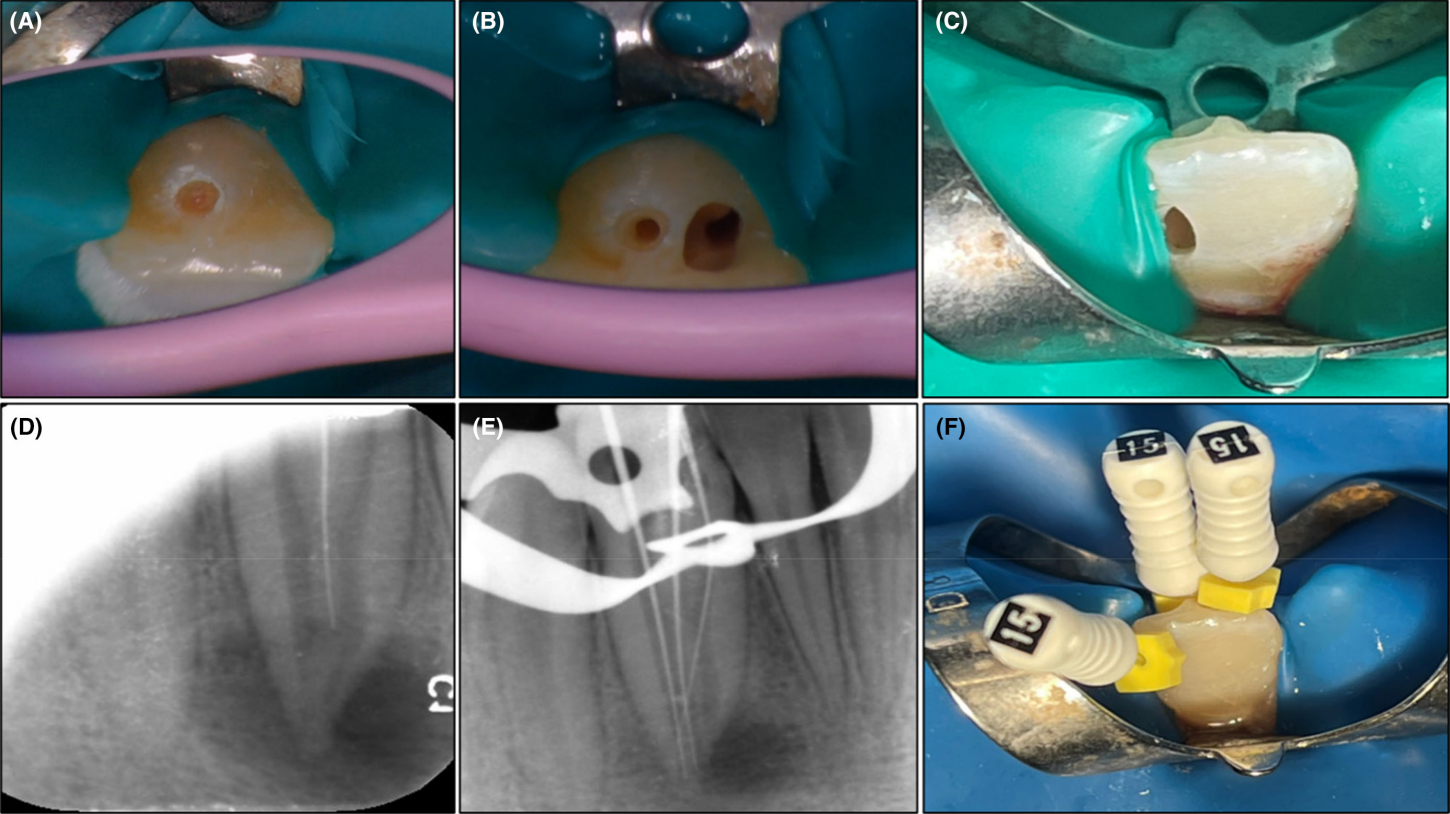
(A) First access cavity in the tip of talon cusp. (B) Second access cavity in distolingual surface of the tooth crown. (C) Third access cavity in mesiobuccal surface of the tooth crown. (D) Exploration of the canals with a #10 C‐pilot file. (E, F) Confirming working length by radiography.
